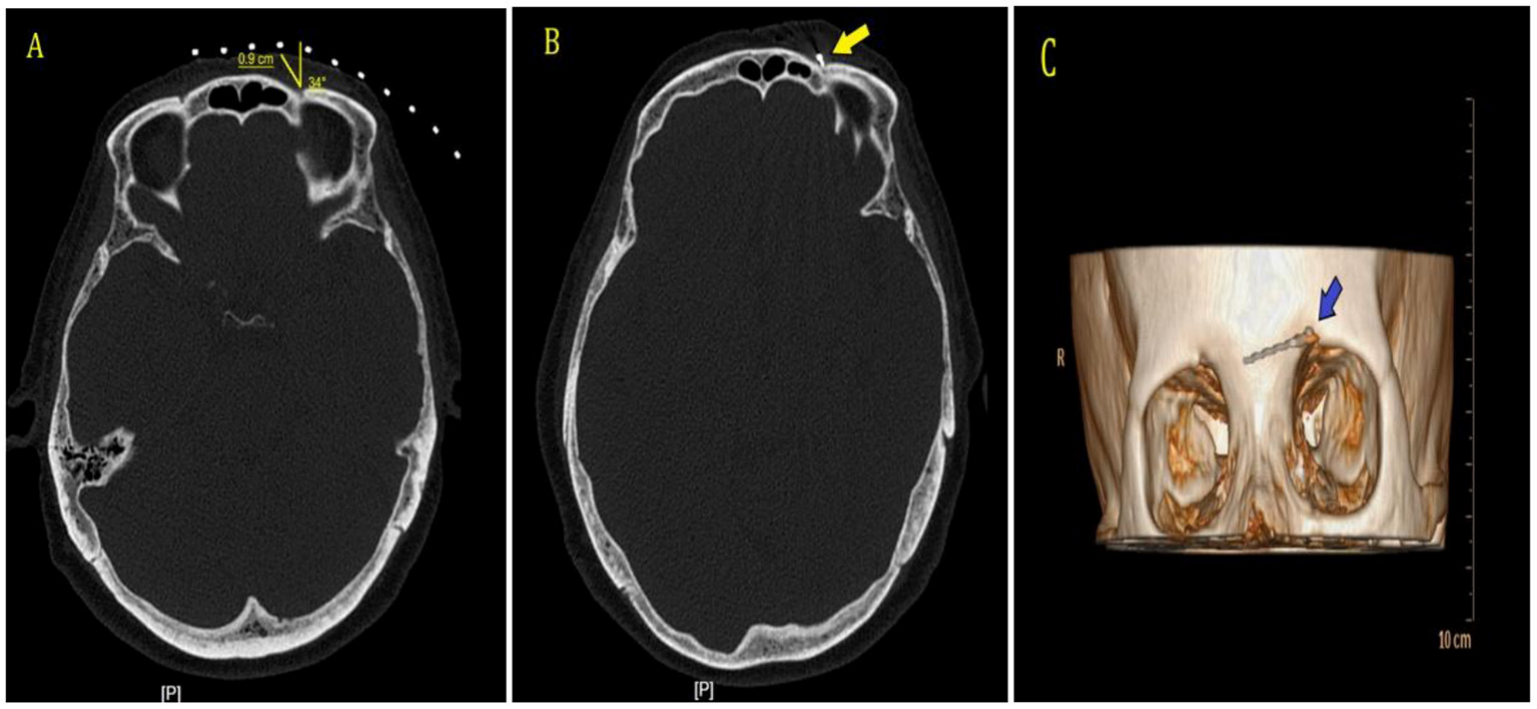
CT guidance and 3D reconstruction. (A) CT scan was performed to establish puncture point and needle path. (B) CT scan showed that the radiofrequency needle was located in the left supraorbital foramen, shown by the arrow. (C) 3D-CT showed the radiofrequency needle was located in the left supraorbital foramen, shown by the arrow.
